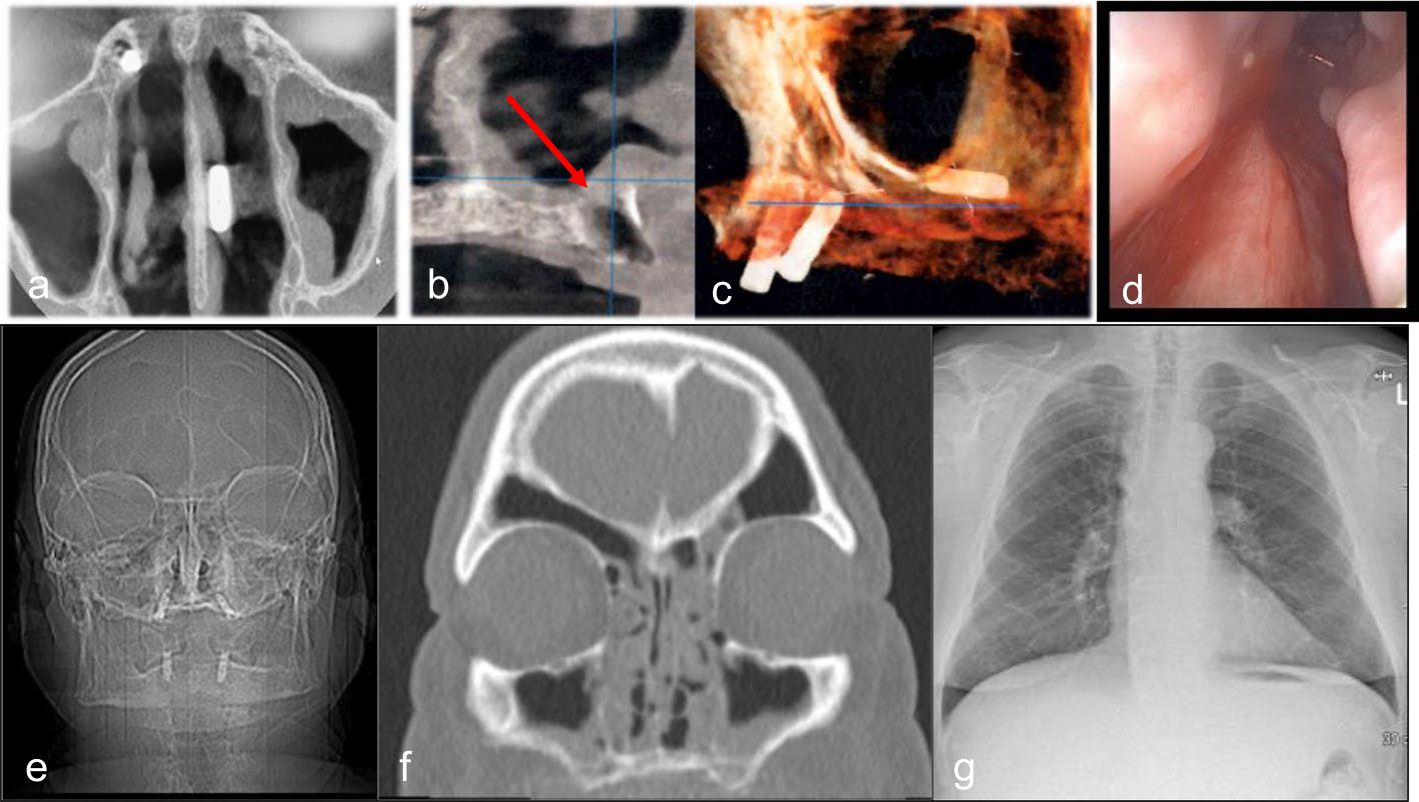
Case 2 - Implant displacement into Nasal Cavity and removal attempt under general anaesthesia the following day. a-c Cone-beam computed tomography (CBCT) taken immediately after displacement revealed the implant on the floor of the nasal cavity, left to the nasal septum. The scan also indicated discrete bilateral mucosal thickening, suggestive of chronic sinusitis. a axial view, (b) coronal view (arrow indicates the bony destruction), (c) volume rendering. d Anterior Rhinoscopy showing protrusion of the posterior nasal floor with slight obstruction of the lower nasal meatus. Lower raw: Postoperative Imaging for Detection of the Migrated Implant: e CT of the paranasal sinuses (PNS): The topogram displays the four osseointegrated implants but does not show the migrated one. f CT PNS coronal view: Features partial opacification of the ethmoidal cells bilaterally. There is wide, concentric mucosal swelling in the left maxillary sinus, along with broad mucosal swelling at the medial wall of the right maxillary sinus and in the septated right portion of the sphenoidal sinus. g Chest X-Ray: Shows no evidence of foreign body aspiration
